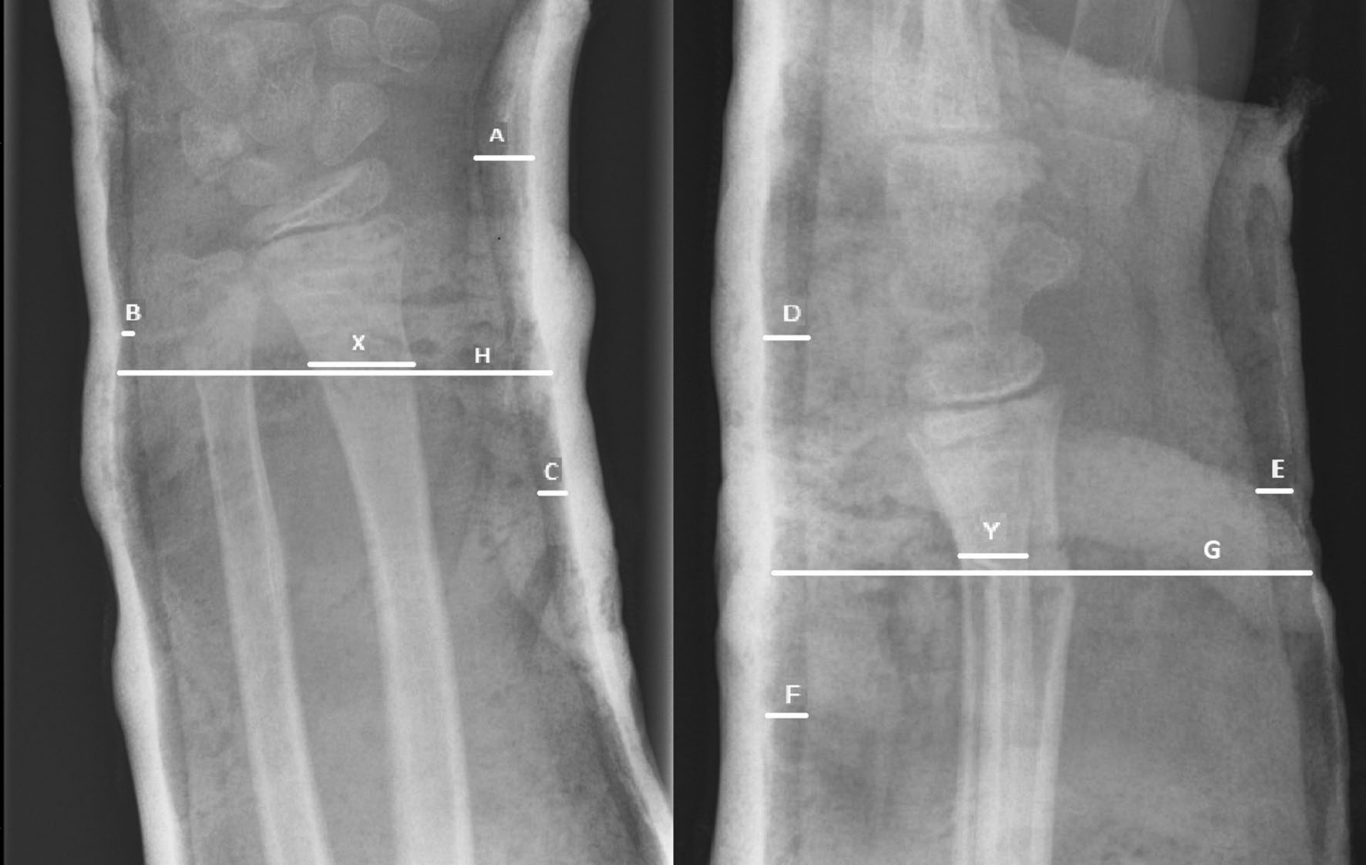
Calculation of the Cast Index and Three-Point Index on the anteroposterior (on the left) and lateral (on the right) radiographs. The Cast Index is defined as the inner cast width at the fracture site on the lateral radiograph (G) divided by the inner cast width on the anteroposterior radiograph (H). The Three-Point Index is defined as [(A+ B+C)/X] + [D+ E+F)/Y] with on the anteroposterior radiograph: A the narrowest radial-side gap between cast and skin around radiocarpal joint or scaphoid; B the narrowest ulnar side gap between cast and skin within 1 cm of the fracture; C the narrowest radial-side gap, 3–5 cm proximal to the fracture side. On the lateral radiograph, D the narrowest dorsal-side gap between skin and cast at radiocarpal joint or proximal carpal row; E and F similar to B and C, however, at the volar- and dorsal-side gap, respectively, on the lateral radiograph [5, 18, 19]
